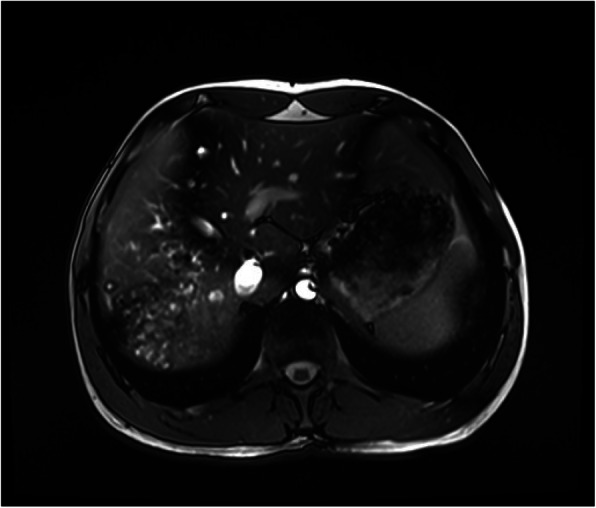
MRI of the abdomen cavity - fluid lesions and disseminated calcifications within V, VI and VII liver segments and widening of the intrahepatic biliary tracts
